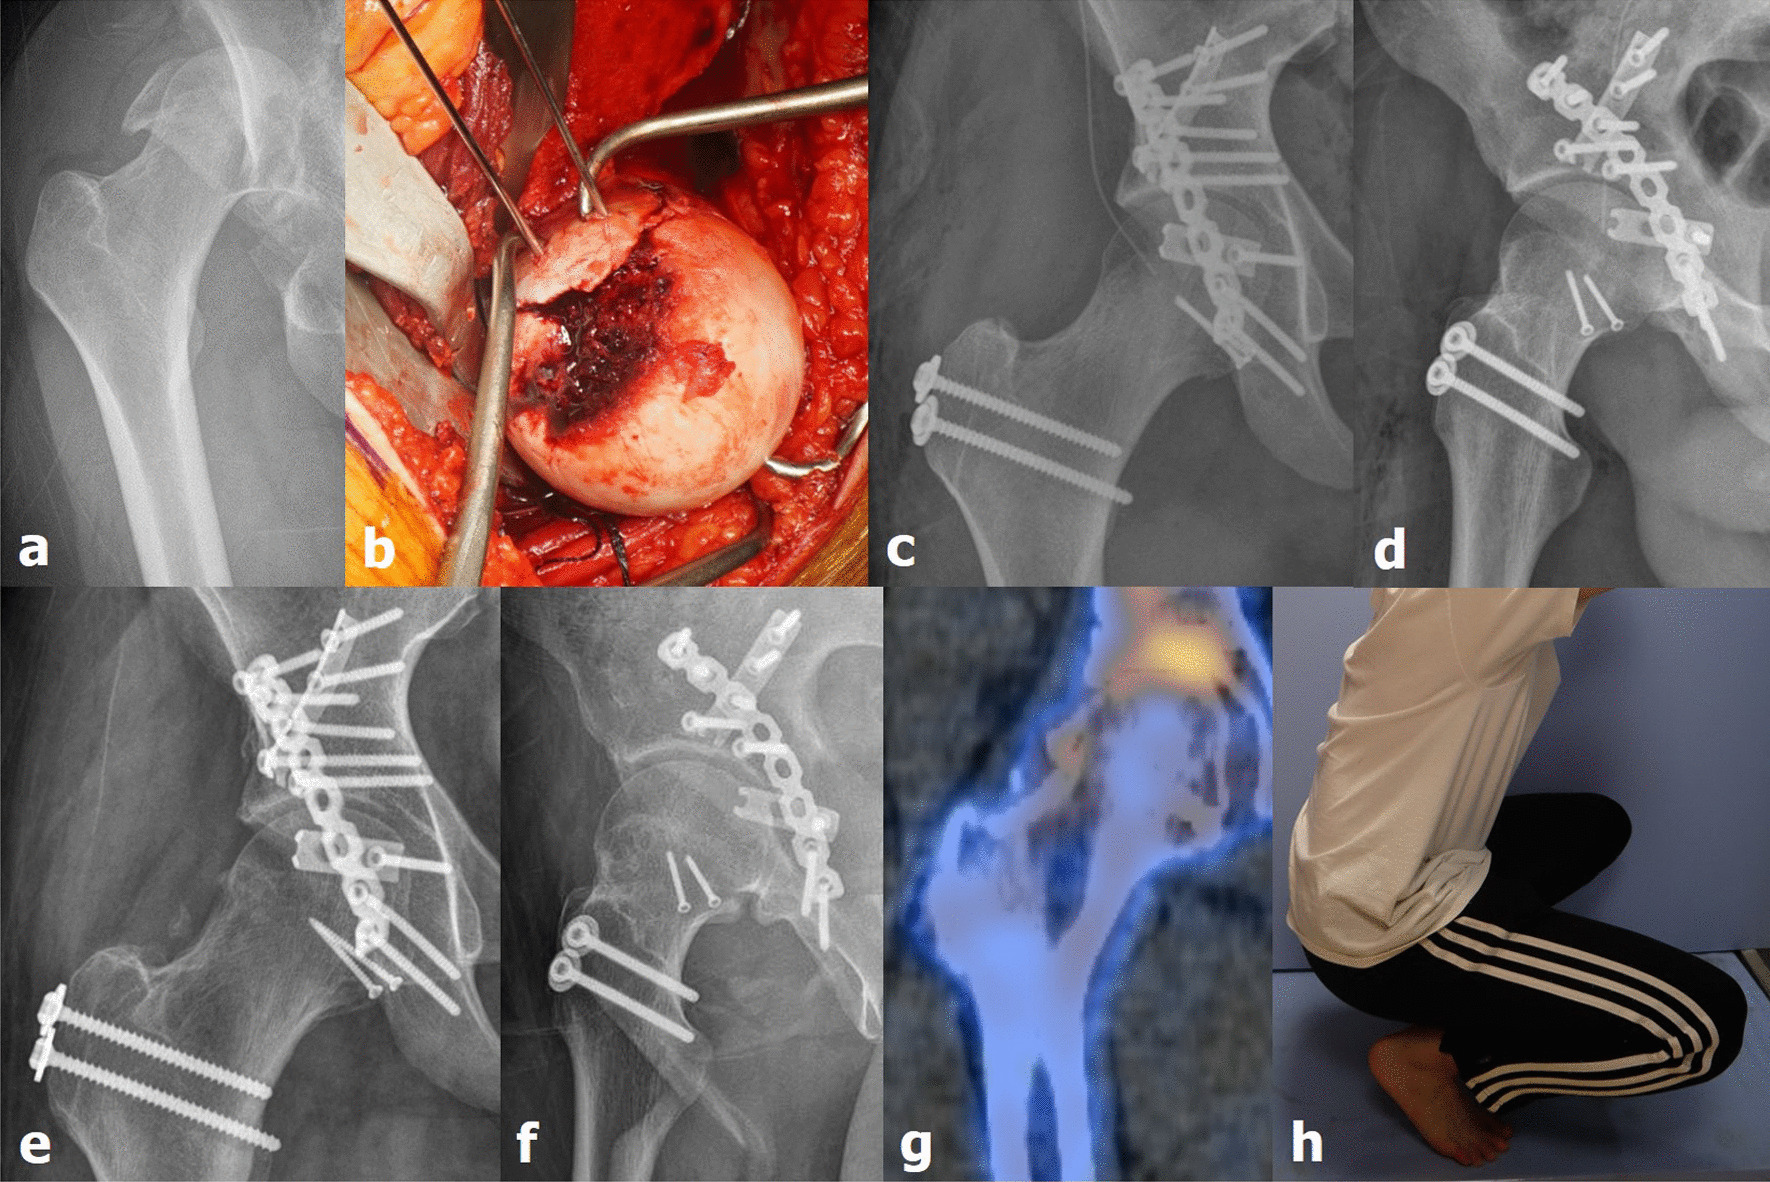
(a) Pipkin type IV femoral head fracture and dislocation of the right hip joint owing to an in-car traffic accident (case 14, a 36-year-old man). (b) Surgical hip dislocation was performed using the modified Gibson approach. The femoral head fracture was reduced using pointed reduction forceps; fixation was performed using 2.7-mm cortical screws. (c, d) Stable fixation was achieved, and good congruence of the femoral head was found to be maintained on postoperative radiography. (e, f) Bone union was achieved 8 months after surgery, and (g, h) blood supply to the femoral head was found to be well maintained on single-photon emission computed tomography/computed tomography, and the patient fully recovered range of motion
